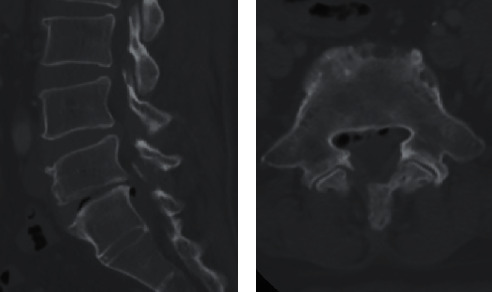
CT scan images revealing multiple gas bubbles at L5/S1 level as shown in axial (a) and sagittal (b) cuts.
